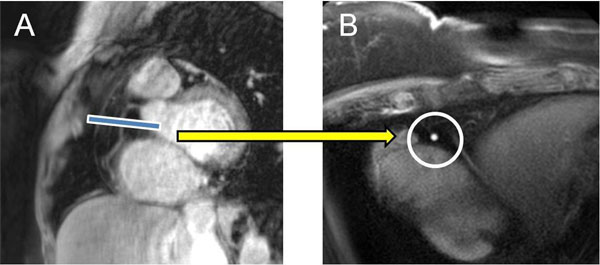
Typical anatomical bright blood coronary images using MRI. In an obese subject with body mass indea of 35, in image A, a scout scan obtained parallel to the RCA (right coronary artery) is shown with the location for cross-sectional imaging (blue line). In B, a corresponding coronary cross section is shown (circled in white) from which area measurements are made before and during isometric handgrip stress.
